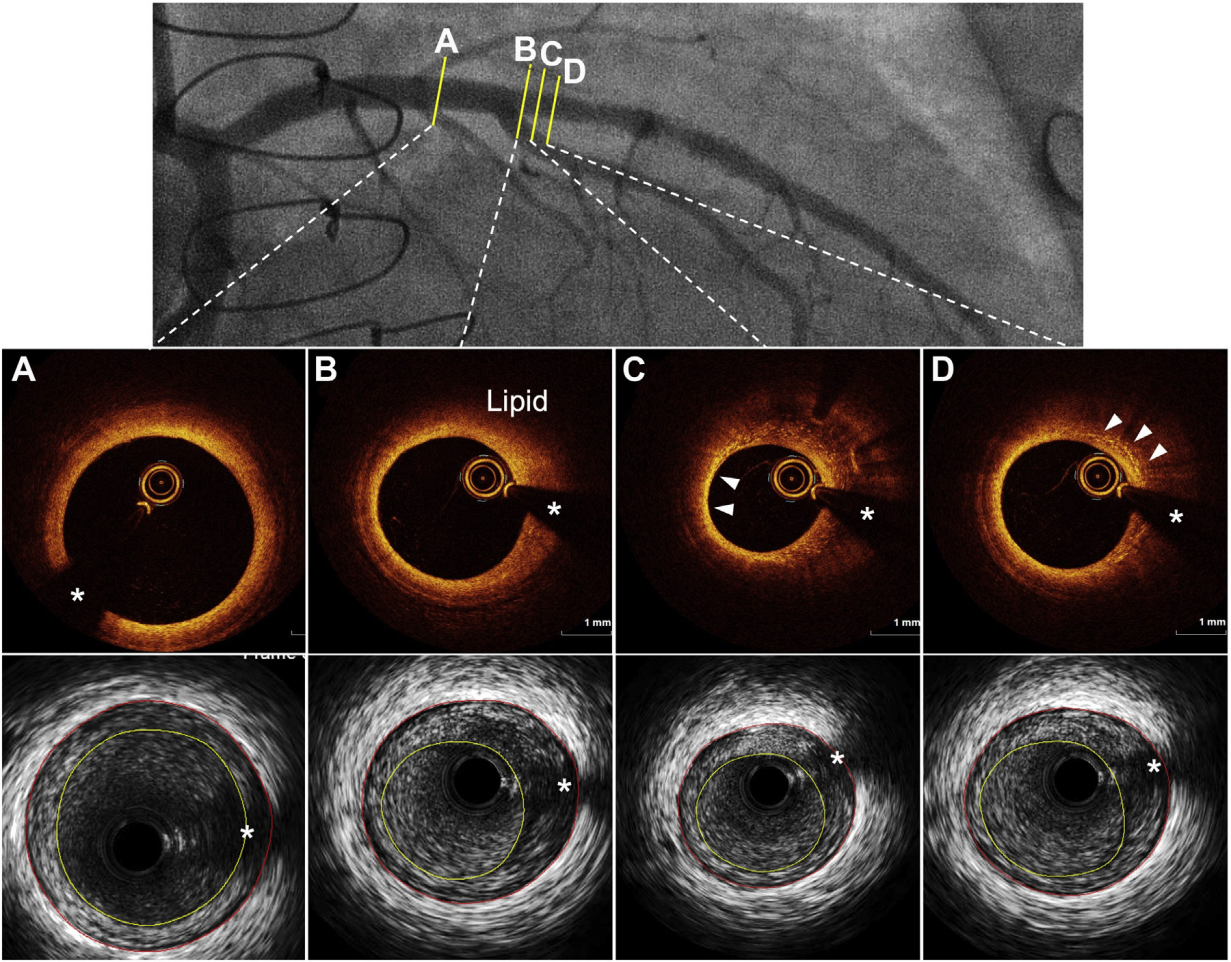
OCT and IVUS images of cardiac allograft vasculopathy. (A) Concentric fibrous plaque, (B) lipid-rich plaque, (C) thin-cap fibroatheroma (arrowheads), (D) accumulation of macrophages (arrowheads). ∗Guidewire artifact. Higher resolution of OCT offers superior delineation of each structure of plaque composition. On the other hand, IVUS can provide deeper visualization of entire vessel wall to assess plaque thickness/area and vessel remodeling. IVUS, intravascular ultrasound; OCT, optical coherence tomography.
